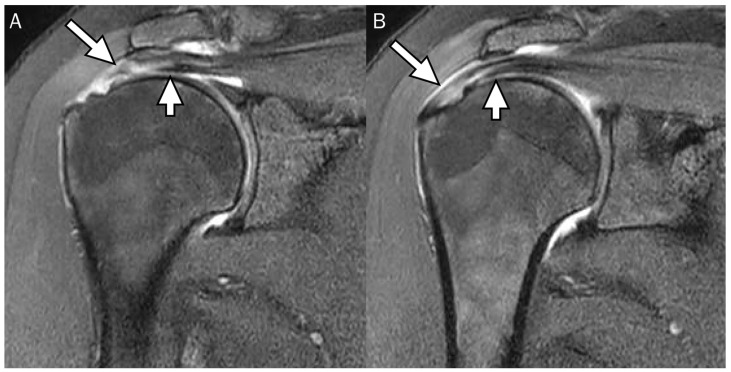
Articular-delaminated full-thickness and partial-thickness tear of the supraspinatus tendon. (A) Oblique coronal fat-suppressed T2-weighted image at the mid-portion of the supraspinatus tendon shows an articular-delaminated full-thickness tear with a visible cleavage and (B) subsequent posterior image of coronal fat-suppressed T2-weighted image demonstrates an articular-delaminated partial-thickness tear with a visible cleavage. Note that the articular surface (short arrows) is retracted more medially than the bursal surface (long arrows).
